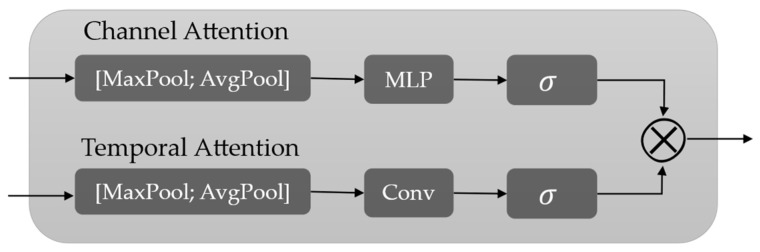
The CTA module structure.
